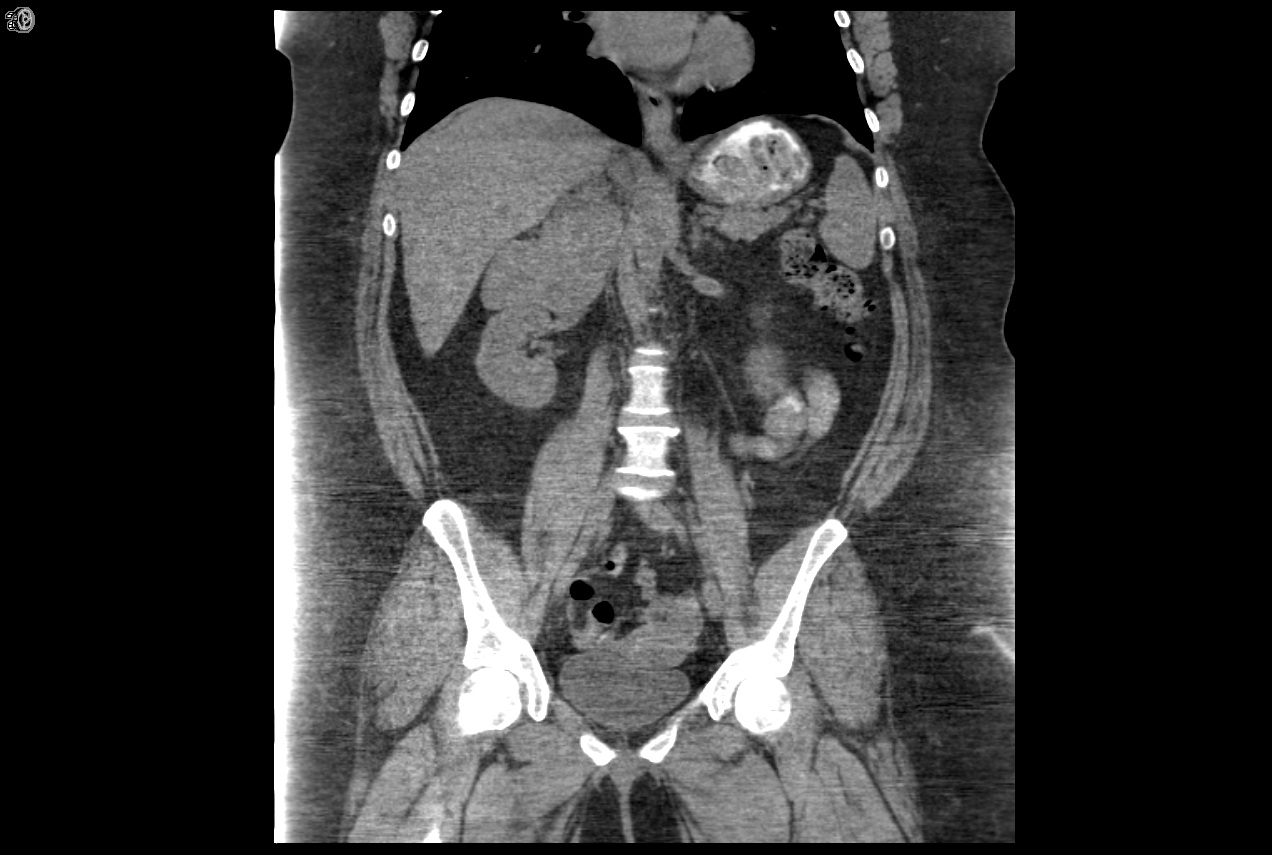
Preoperative CT of the abdomen and pelvis (coronal view) showing multilobulated mass involving the right adrenal gland.
